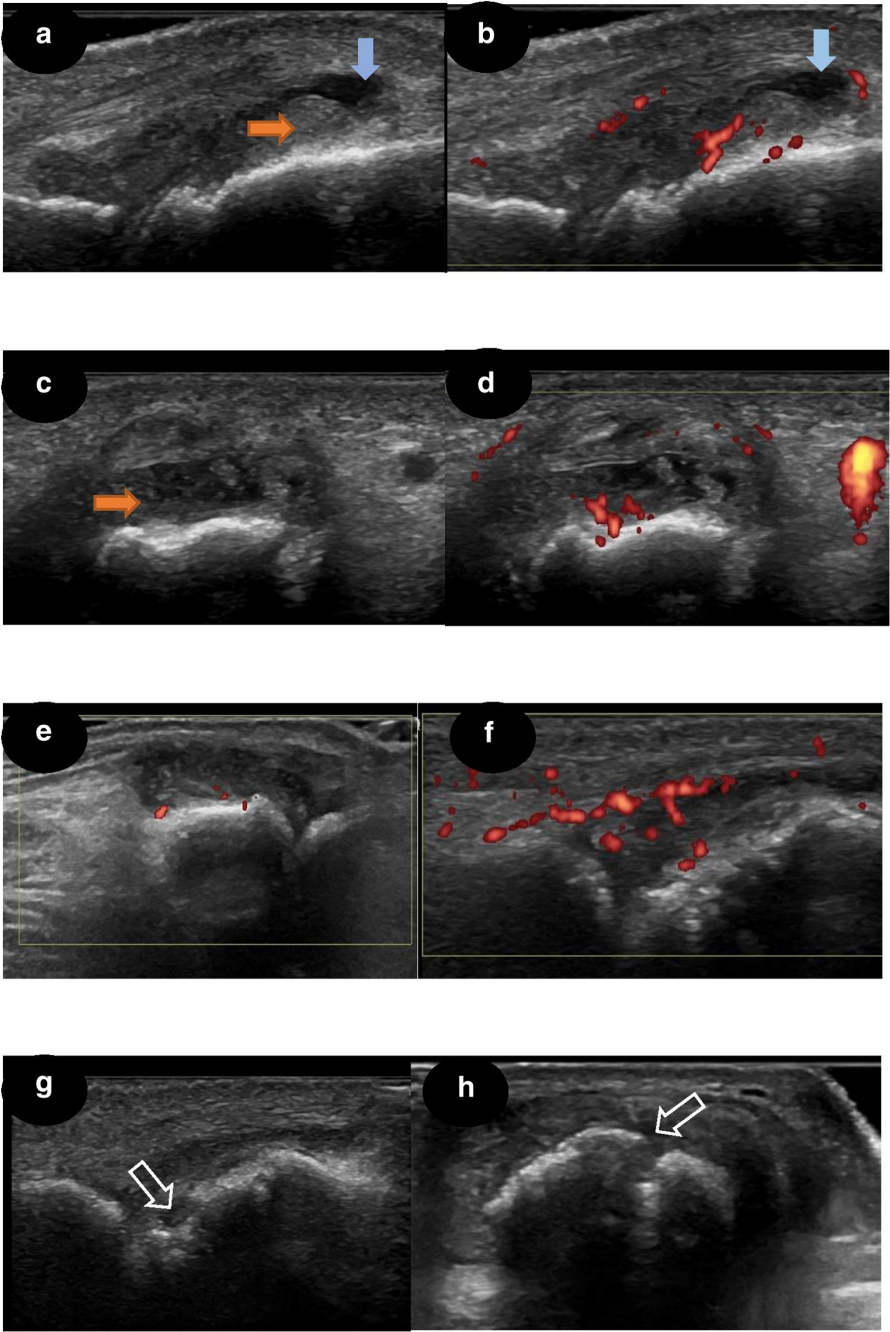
Examples of US grading. a–d MCP joint with effusion, SH grade 3 and PD grade 2, longitudinal and transversal views. e PIP joint with SH grade 3 and PD grade 1. f PIP joint with SH grade 2 and PD grade 3. g, h PIP joint with erosion, longitudinal and transversal views; white arrow, erosion; orange arrow, SH; blue arrow, joint effusion; SH, synovial hypertrophy; PD, power Doppler signal
